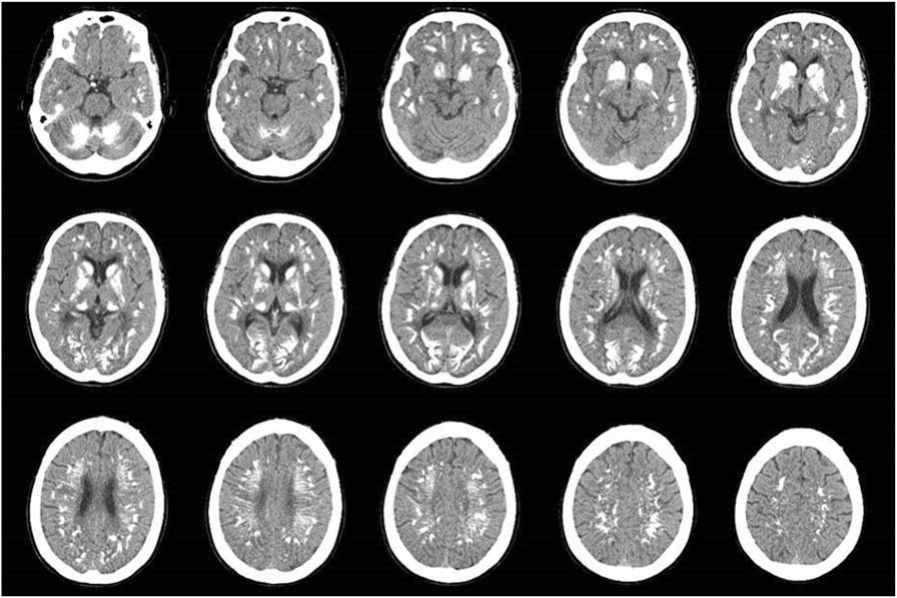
Brain computed tomography scan after generalized tonic-clonic seizure shows multiple symmetric calcifications of the basal ganglia, cerebral cortex, and cerebellum
